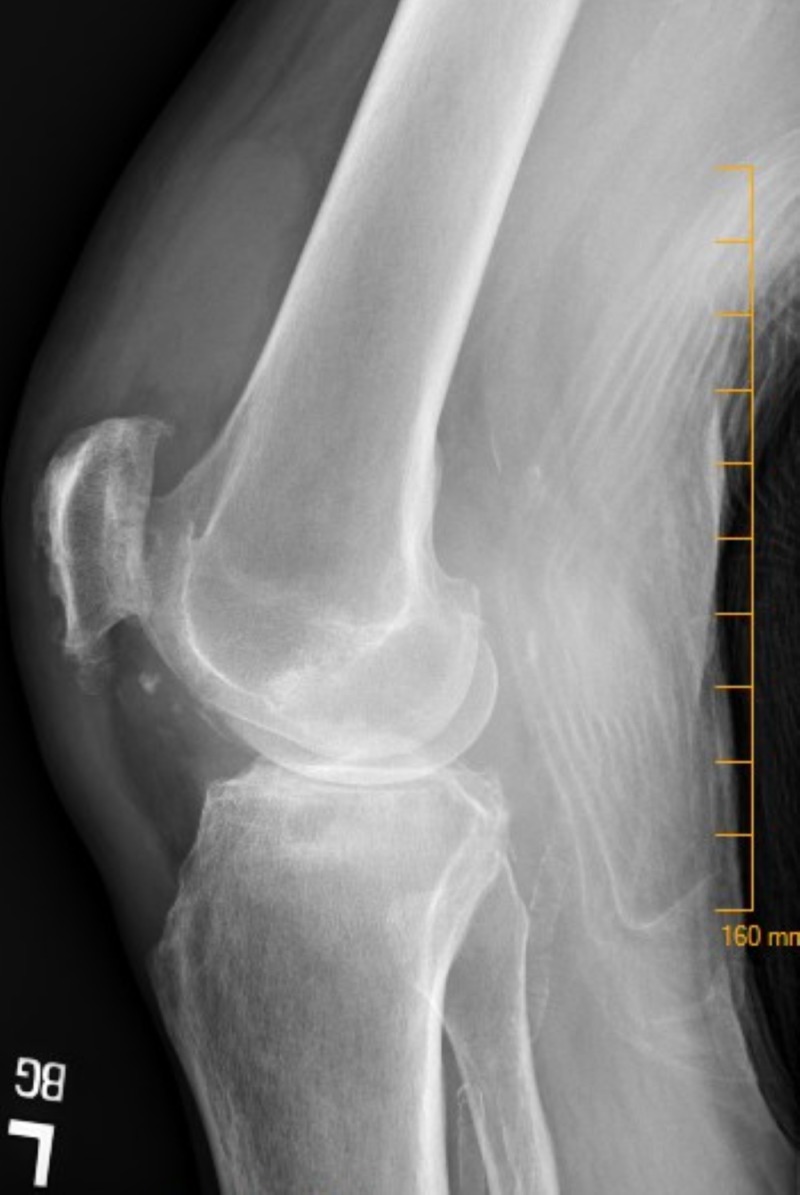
Left Knee The figure depicts a left knee x-ray demonstrating osteoarthritis and a substantial joint effusion.
